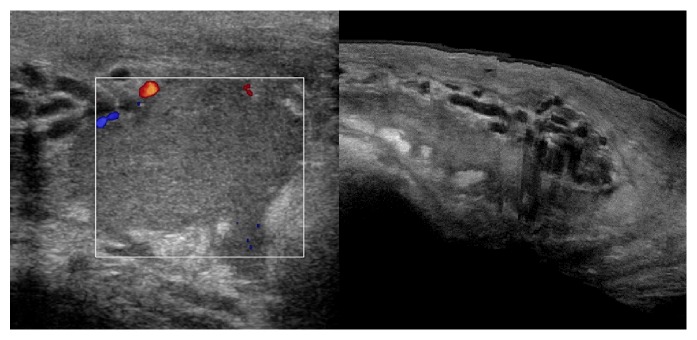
Doppler ultrasonography. Left: a well-defined area with heterogeneous echotexture on the left extratesticular tissue. No vascularity was noted in the lesion. Right: topography of the hematoma related to the varicocele. Scrotal wall thickening is also noted.
